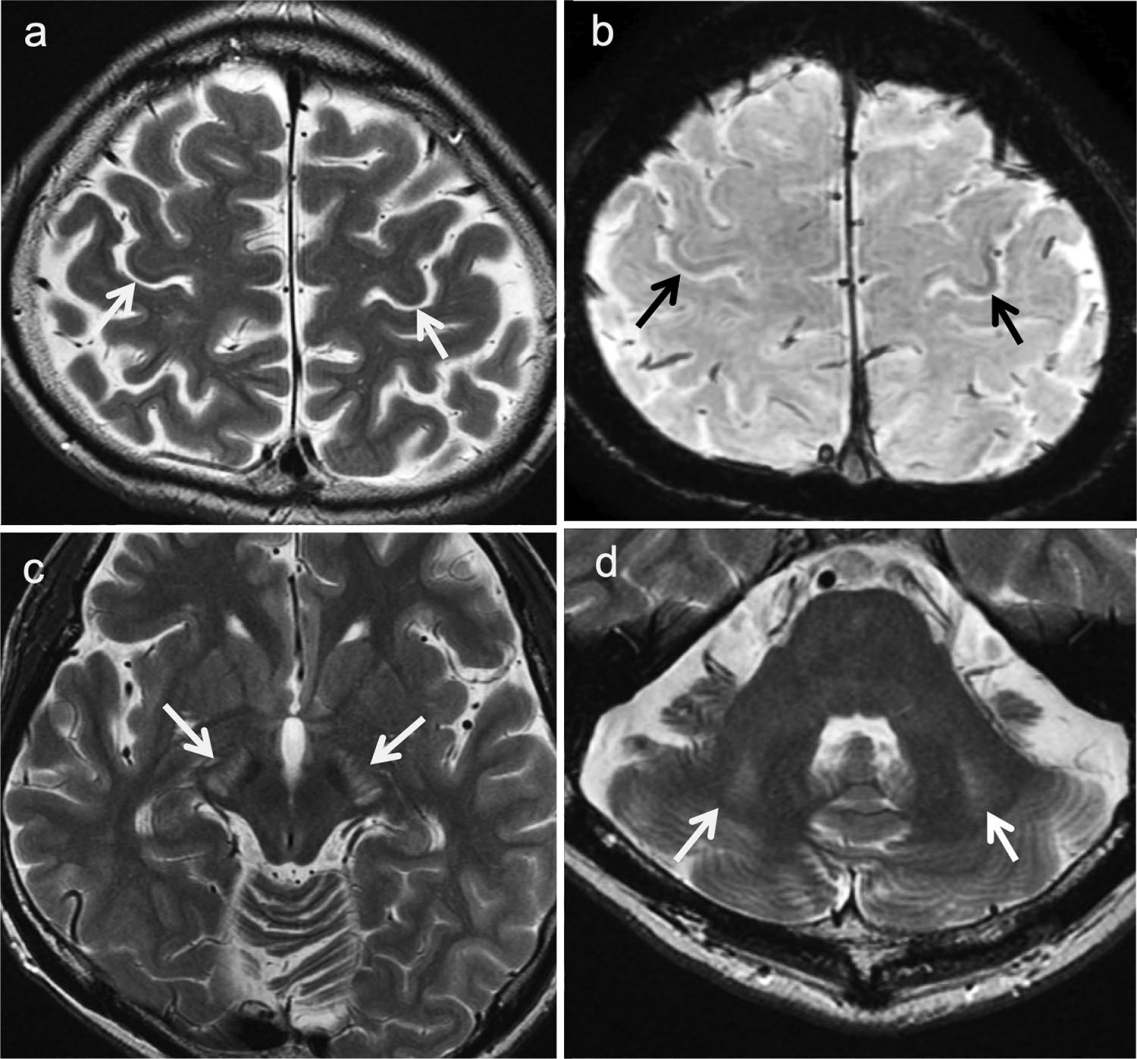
Citrullinemia type II (CTLN2) (chronic progressive type). A 42-year-old, male patient with epileptic seizures. The patient had hyperammonemia and hypercitrullinemia with normal liver function. a, b T2-weighted images (a) and SWAN (b) displayed bilateral, low signals in the deep, bilateral, precentral cortices (arrows). c, d T2-weighted images demonstrated hyperintensity in the bilateral cerebral peduncles and middle cerebellar peduncles (arrows). (Source: reference 21)
